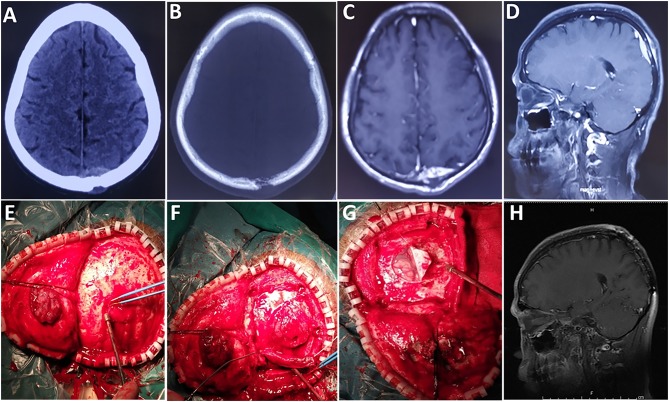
A case of epidural tumor. (A,B) Preoperative CT scans show that the lesion had broken through the outer table of the skull to invade the scalp. (C) Contrast-enhanced axial and (D) sagittal images show significant enhancement. (E,F) The tumor was reddish and soft, with an abundant blood supply. (G) The tumor did not invade brain tissue. (H) Images obtained 6 months after surgery demonstrated no local tumor recurrence.
